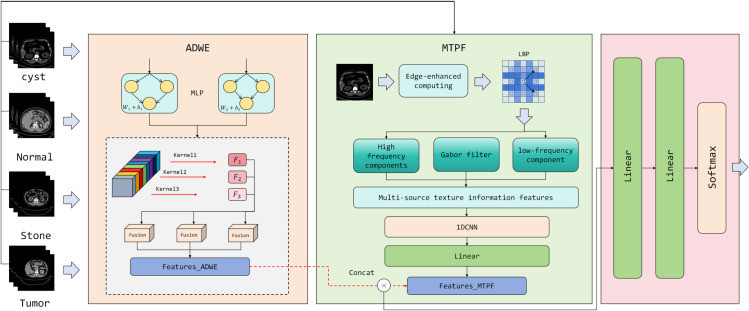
Overall framework of the proposed kidney CT classification model. The ADWE module adaptively enhances contrast through dual-window fusion, while the MTPF module integrates edge, LBP, and Gabor priors for multi-scale texture representation, and the fused features are fed into the classifier for final prediction.
